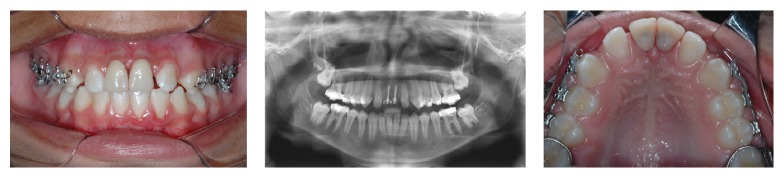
Clinical and radiographic aspects 6 months after mini-implant placement.
